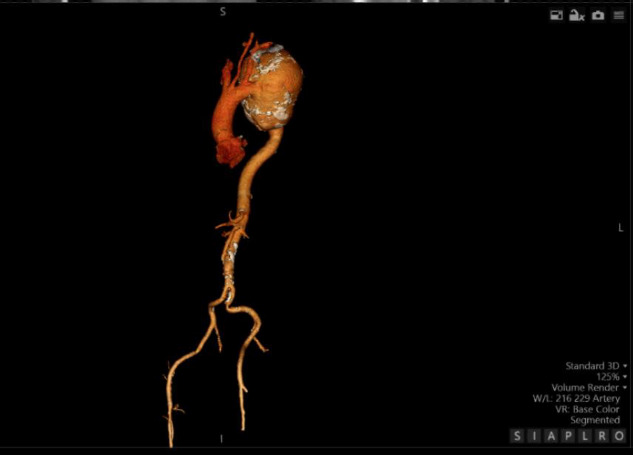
Detailed 3-D CT scan of aortic arch and the rest of the aorta
